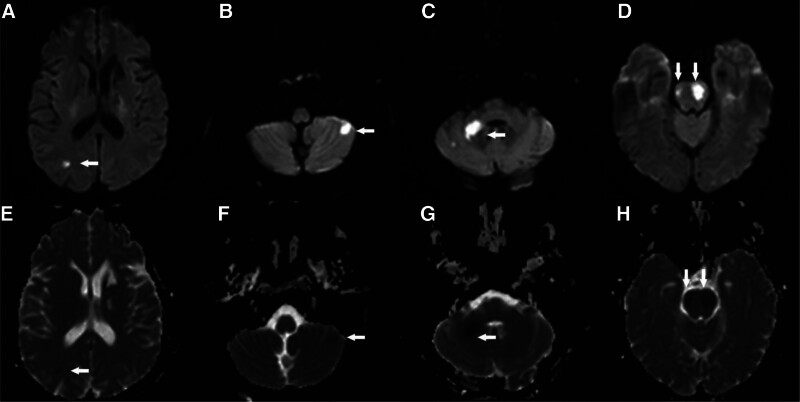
(A–D) Acute cerebral infarction on DWI sequence (white arrow). (E–H) Low signal on the corresponding ADC sequence (white arrow). ADC = apparent diffusion coefficient.
